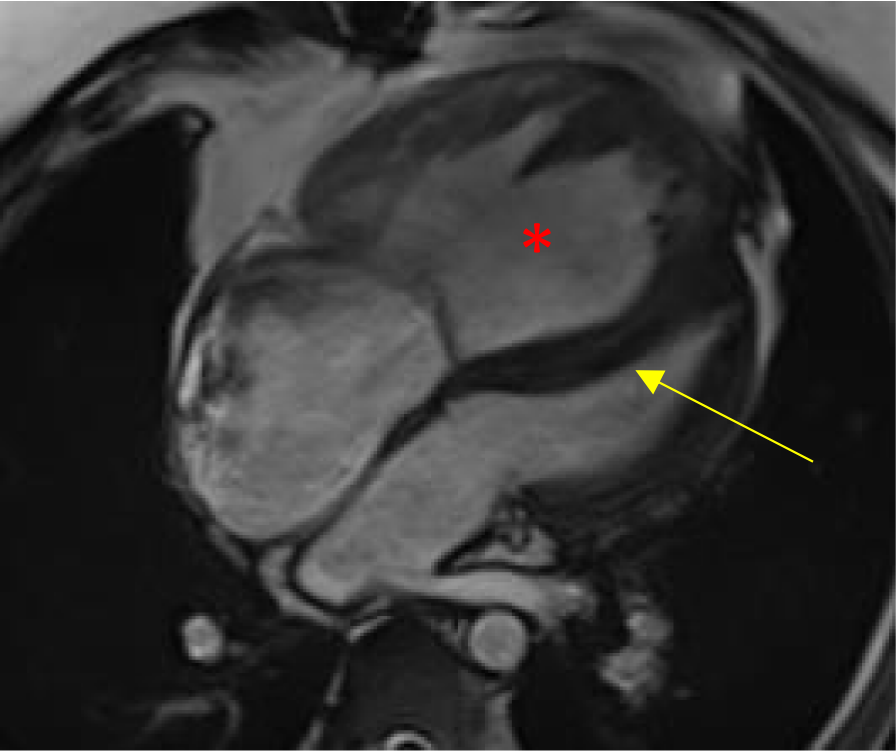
Four-chamber MRI SSFP cine in a patient post-TOF repair. There is significant right ventricular hypertrophy and dilatation (red asterisk). The interventricular septum is also shifted towards the left ventricle (yellow arrow)
